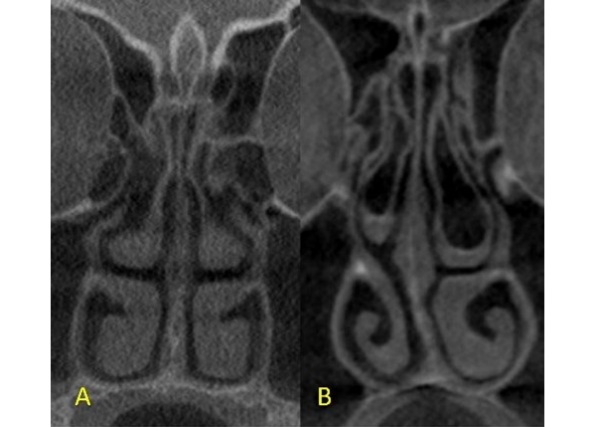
Cropped coronal cone beam computed tomography images showing (A) a nondeviated nasal septum and (B) a deviated nasal septal deviation.
